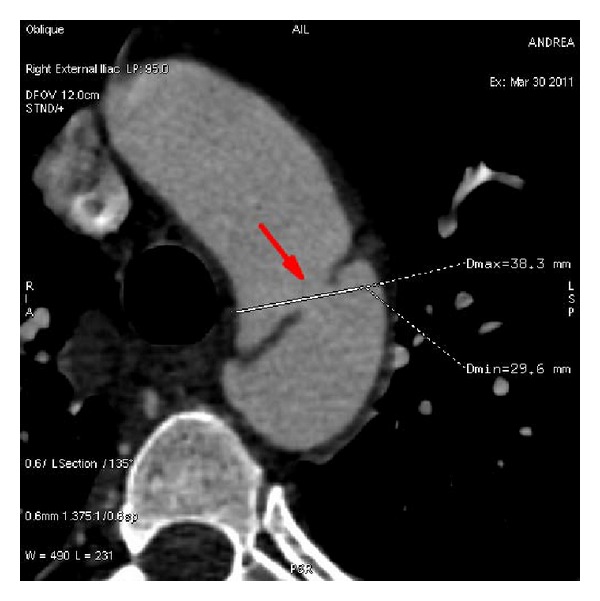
CTA image shows the location of tear in thoracic aorta (arrow).
